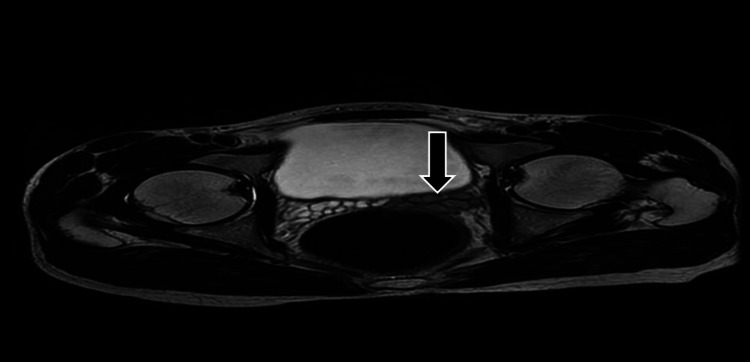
Magnetic resonance imaging (axial T2) showing hypointense cysts on left seminal vesicles (arrow)
